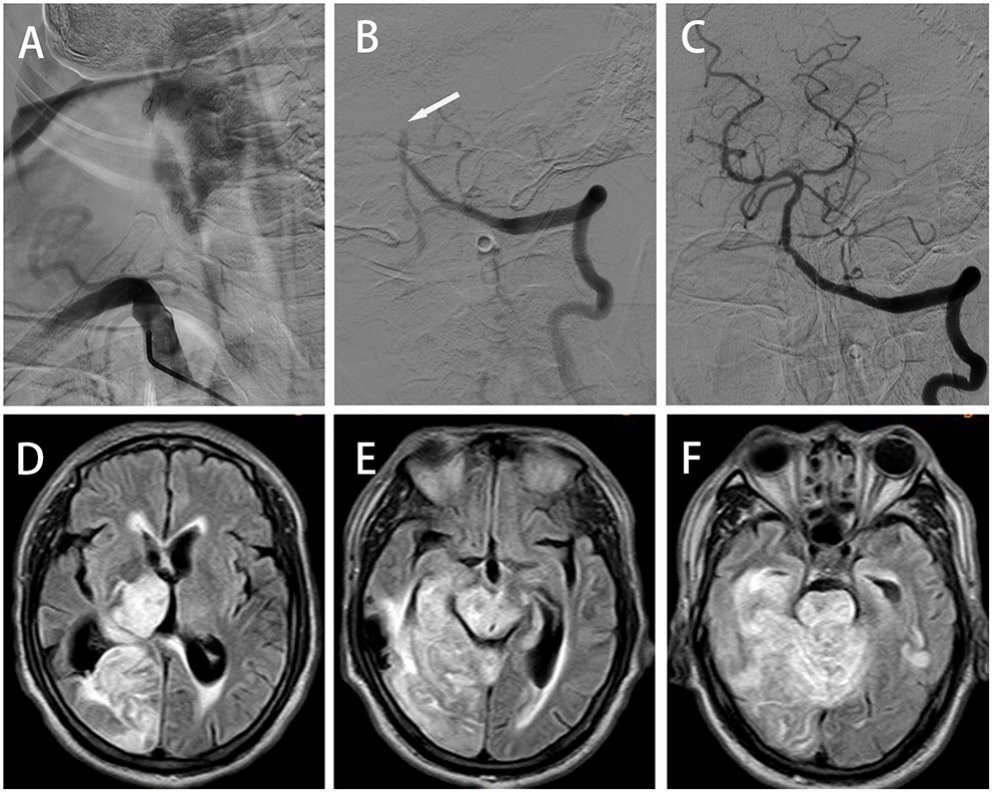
Case 1: (A) Anterior–posterior (AP) digital subtraction angiography (DSA) showing occlusion at the beginning of the right vertebral artery (VA). AP left VA angiogram demonstrating basilar artery occlusion ((B), arrowheads); successful recanalization was obtained by endovascular thrombectomy (C). (D–F) Axial FLAIR magnetic resonance imagining (MRI) showing multiple infarction lesions, involving the right thalamus, corpus callosum, right occipital lobe, right temporal lobe, right cerebellar hemisphere, cerebellar vermis, midbrain and pons.
